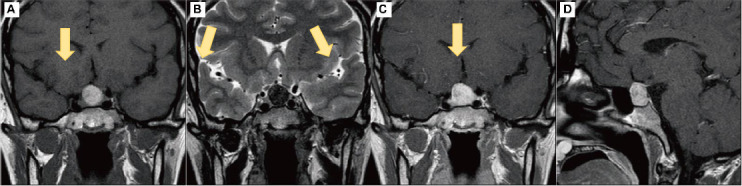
Preoperative Pituitary MRI Plain and Enhanced Scans. (A) Coronal MRI T1-weighted and (B) T2-weighted images show a pituitary lesion with an unclear pituitary stalk and an enlarged sella turcica (as indicated by the arrow). (C) Coronal and (D) sagittal T1-enhanced images display mild enhancement with small patchy non-enhancing areas within the lesion (as indicated by the arrow).
